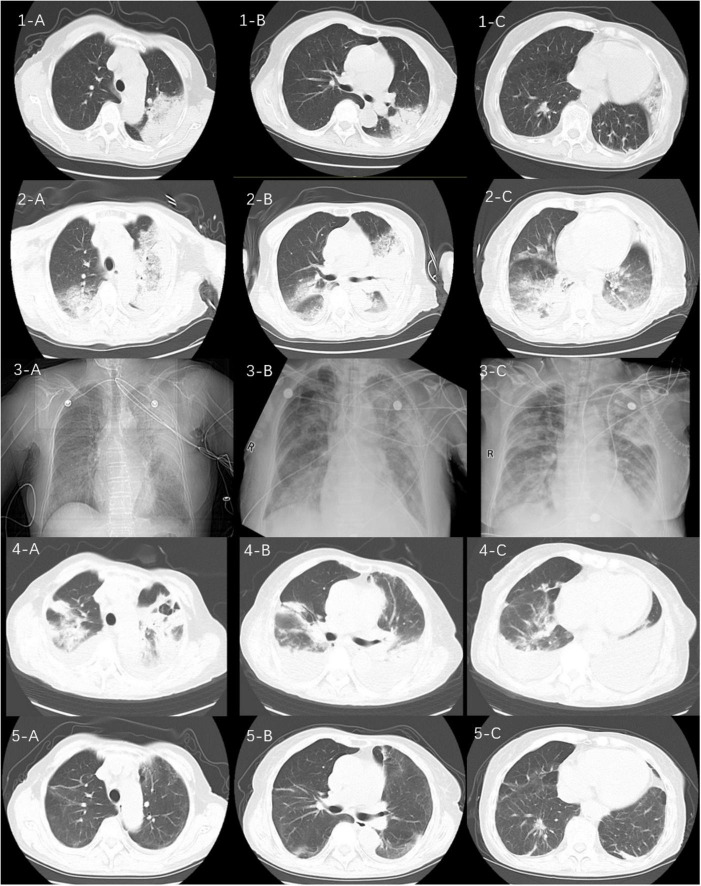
Sequential computed tomograms (CTs) of the patient’s chest. CT at a local hospital (1A–C) appeared to show a solid shadow in the upper lobe of the left lung. CT on admission (2A–C) showed multiple exudative lesions in both lungs. The next images (3A–C) represent the patient’s chest x-ray findings at the time of admission to our hospital, on the 5th and 10th days, respectively. CTs on the 15th day of admission (4A–C) suggested infection in both lungs with partially absorbed and partially enlarged lesions as well as an enlarging pleural effusion on the left side. CTs 1 month after discharge (5A–C) show substantial resorption of the lesions in both lungs.
